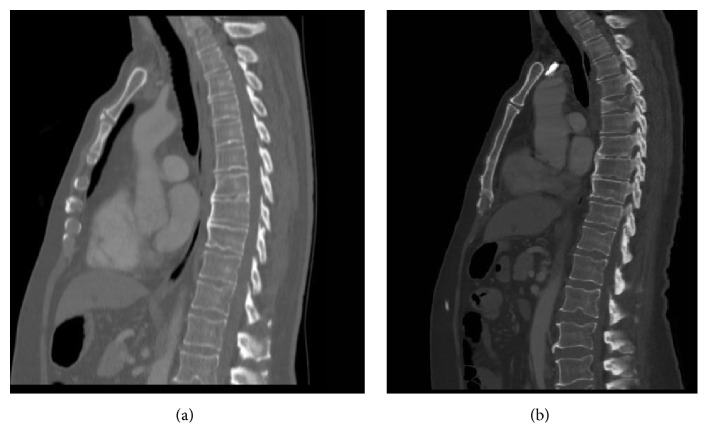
(a) CT scan at the time of penile resection. (b) CT scan at the time of A+E presentation with weakness.
